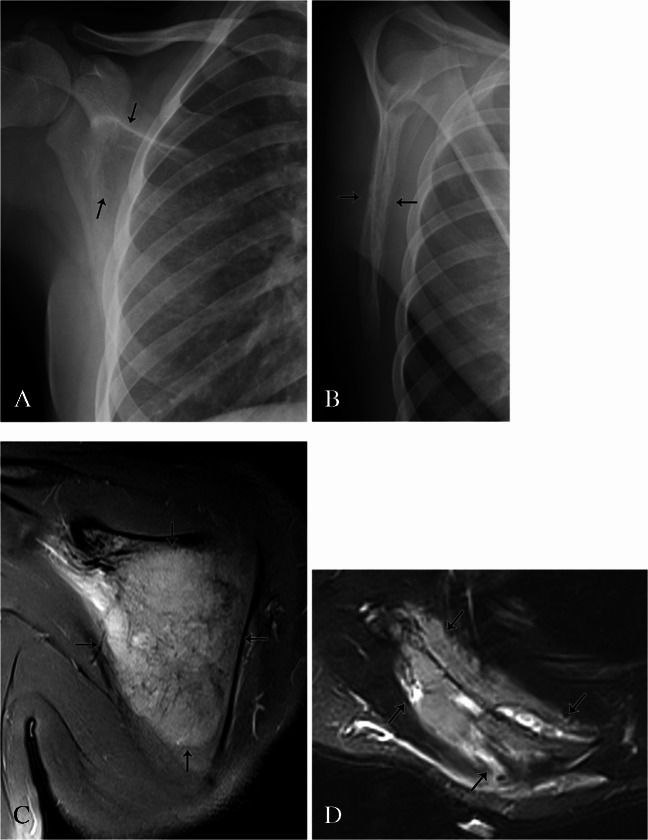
12-year-old female with Ewing sarcoma of the right scapula. AP (A) and lateral (B) radiographs demonstrate a permeative lytic lesion (arrows). Coronal (C) and axial (D) proton density (PD) fat saturation (FS) MRI images show significantly more extensive involvement than suggested on x-ray, as well as a considerable extra-osseous extension (arrows)
